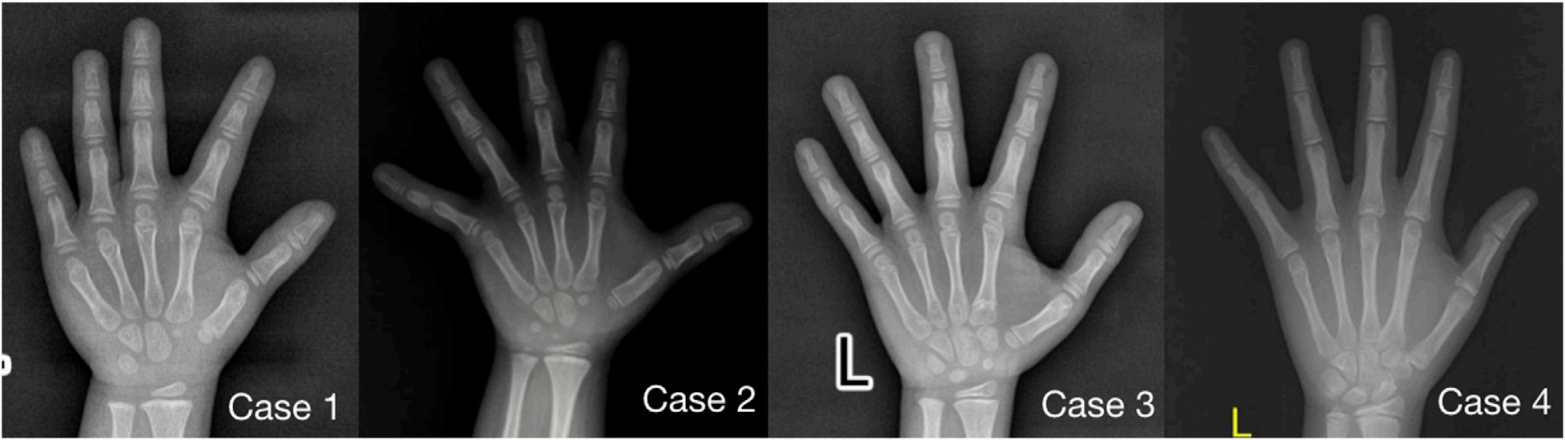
Anterior-posterior radiographs of the metacarpal and phalangeal bones of four children with 3M syndrome. The picture of Case 1 was taken at 5.3 years old, without obvious slender changes of tubular bone, and the BA was 4.0 years old; Case 2 was taken at the age of 5.3 years old, with slender tubular bone and BA basically consistent with CA; Case 3 was taken at 11.3 years old, the tubular bone was slender and the BA was 8.5 years old, significantly younger than CA; Case 4 was taken at 10.7 years old, with slender tubular bone and BA was 11.9 years old, significantly elder than CA.
